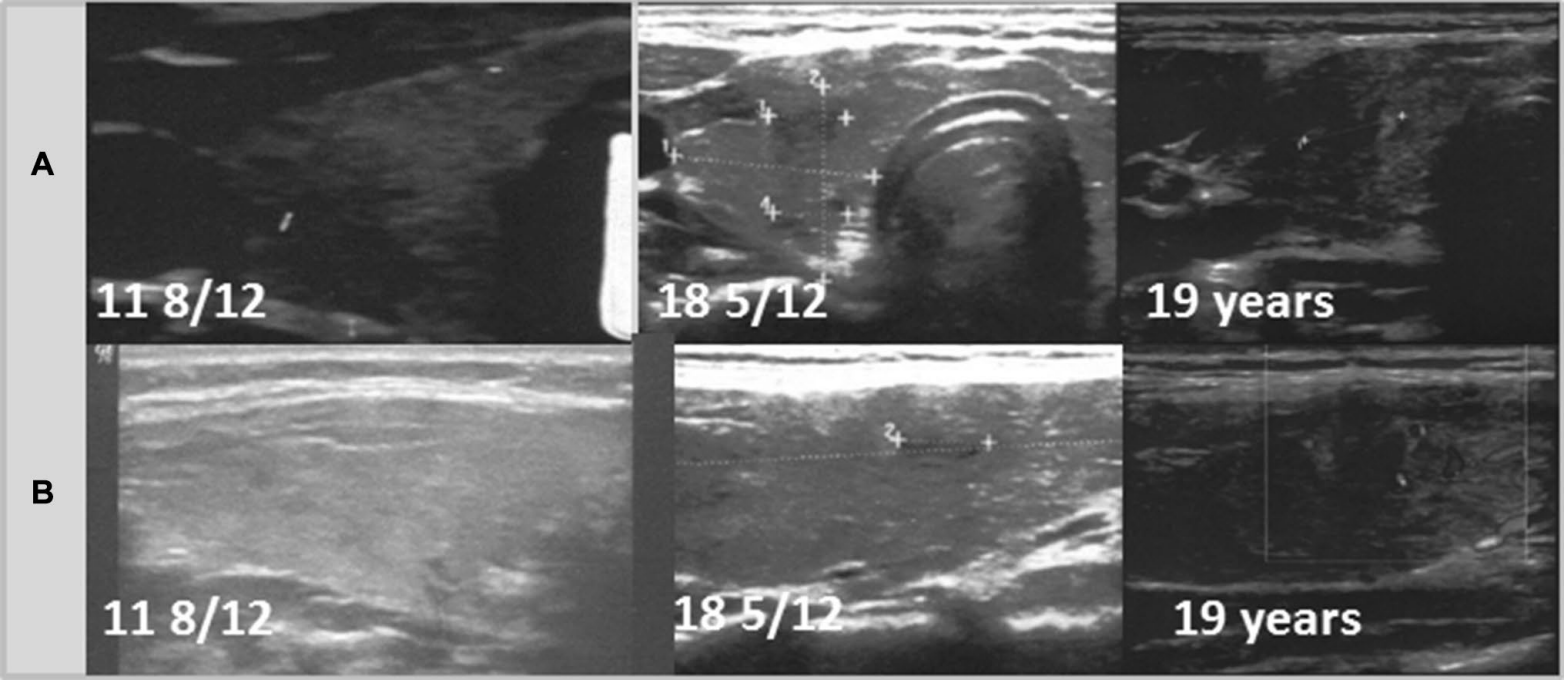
Patient 6. Transversal (a) and longitudinal (b) scans of the normoechogenic thyroid gland revealed formation of the nodule 6 9/12 years since referral and since last ultrasound assessment. The growth of the nodule was fast from 6.3 × 7.3 × 4.2 mm to 12.2 × 7.4 × 8.9 mm in 7 months (316% increase). The nodule was surrounded by a hyperechogenic border
